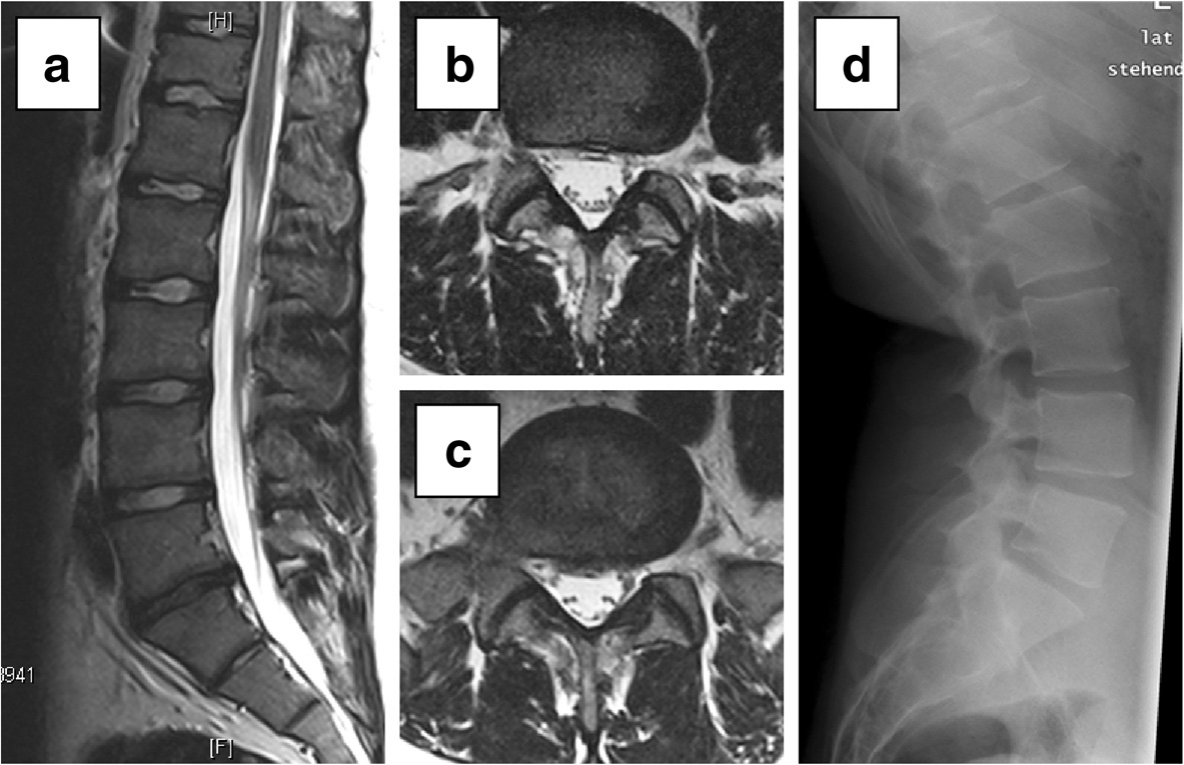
Recommendation for conservative treatment. a-c T2-weighted magnetic resonance images preoperatively: a lumbar sagittal image and b axial lumbar image of L4/5 and c L5/S1. d Lateral radiographic image. The patient presented with predominantly chronic lumbar back pain since childhood and intermittent bilateral sciatic pain. The patient works as a storeman. Advanced intervertebral disc degeneration was observed between L5 and S1 (Pfirrmann grade 4) and mild facet joint degeneration of L4/5 and L5/S1 (Weishaupt grade 1). Before inpatient gradual diagnostics (IGD), a lumbar fusion of L5/S1 with transforaminal lumbar interbody fusion had been discussed. Results obtained during IGD: • epidural injection of L5/S1: 0 % improvement of sciatic pain, 30 % back pain relief. • bilateral facet joint infiltration of L5/S1: 0 % improvement of back and sciatic pain. In view of the lack of response to the infiltrations, further conservative strategy was recommended. Half a year later, the patient had a discectomy of L5/S1 performed in another hospital, with initial good results for a few weeks, followed by a complete clinical relapse. When the patient thereupon presented himself in the department of neurosurgery of our hospital, our colleagues advised against further surgery, independent of our own recommendation
